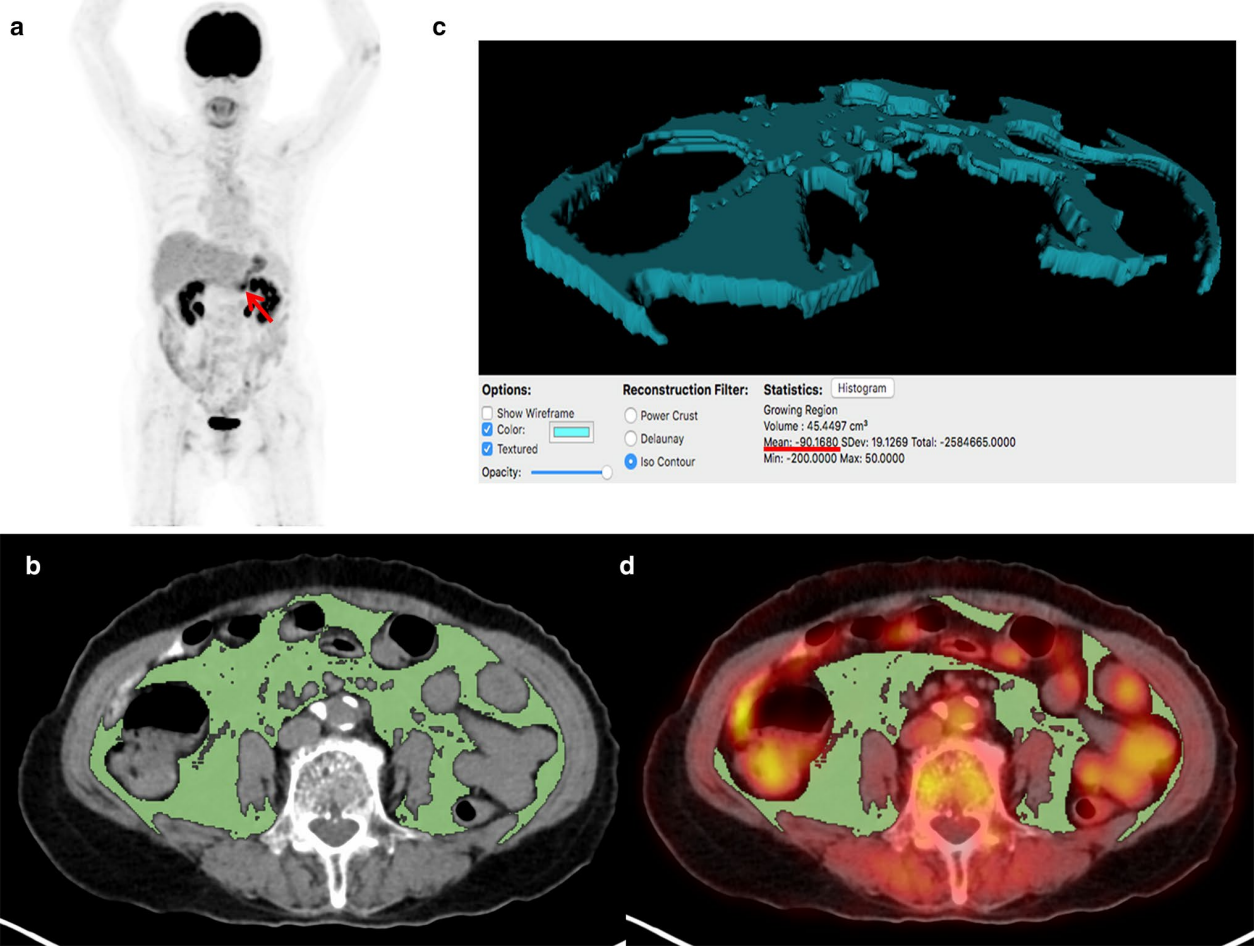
Measurement example of CT attenuation and SUV of VAT. A 79-year-old woman underwent FDG PET/CT for staging work-up of AGC, showing focally increased FDG uptake at lower body of the stomach on maximal intensity projection image (arrow) with SUVmaxT of 4.12 (a). On three consecutive transaxial CT images at the L4-5 spine level, the area of VAT defined as an intra-abdominal area with CT attenuation range between − 200 and − 50 HU was automatically delineated using OsiriX MD 10.0 software (b). With the areas of VAT on CT images, three-dimensional structure of VAT was automatically created and mean attenuation of the structure was calculated, which was − 90.17 (c). Afterwards, the VAT areas on CT images were exported to the corresponding fused PET/CT images. After the removal of FDG activity of the urine, bowels, and vessels, mean SUV of the areas was measured, and was found to be 0.49 (d). The patient underwent subtotal gastrectomy and was diagnosed with pT2N1 stage tubular adenocarcinoma. The patient experienced peritoneal recurrence 10.4 months after the surgery and died 13.3 months after operation
